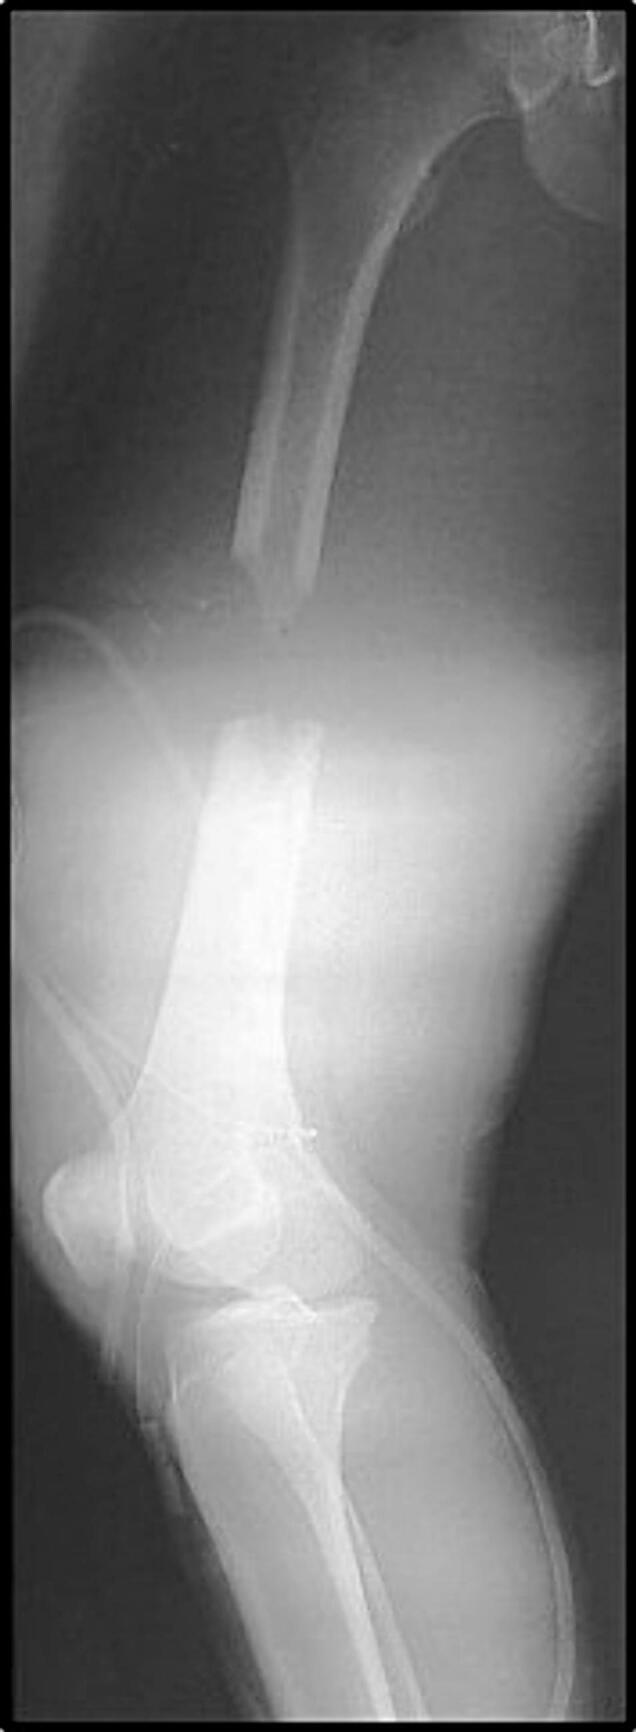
Radiograph showing the circumferential 7-cm defect after Gustilo-Anderson type IIIA femoral shaft open fracture in a 22-year old male patient.
